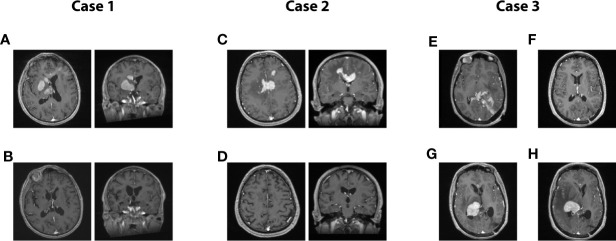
Representative cases from the series. (A, B): Case 1. A 71-year-old man presented with rapidly progressing left-sided hemiparesis combined with a sudden decline in cognitive functioning. On T1-gadolinium enhanced MRI, multiple nodular enhancements are obvious, devoid of significant oedema. Local mass effect can be observed on the ventricular system in axial and coronal acquisitions (A). Same MRI acquisition in axial and coronal after eight cycles of treatment (B). This patient remains disease-free to this date, 18 months after the conclusion of his last cycle. Clinically, he presented a full recovery. (C, D): Case 2. A 62-year-old man presented a gradual deterioration in cognitive functioning over a 2 months interval. Significant slowness in motor initiation and working memory were prominent features on presentation. A T1-enhanced MRI scan revealed multiple nodular enhancing lesions, mostly centered on the corpus callosum, and accompanied by oedema, in axial and coronal (C). T1-enhanced MRI scan in axial and coronal acquired at the conclusion of the treatment plan of 12 cycles, displaying a complete radiological response which was paralleled by a full clinical recovery (D). The patient remains disease-free 7 years after the conclusion of his treatment plan. (E–H): Case 3. A 42-year-old woman presented with mixed aphasia, confusion and severe headaches. What was considered confusion in retrospect was entirely attributable to a receptive speech deficit. She underwent an MRI scan which revealed a diffuse T1-enhancing mass localized in the left parieto-occipital area and diffusely infiltrating the splenium. The mass was partially resected in another center, before she started treatment with our team. The pre-treatment axial T1 MRI is shown in Panel (E), while the post-treatment MRI at the conclusion of eight cycles is shown in Panel (F). The patient was in complete response and had presented a full clinical recovery. She hence decided to stop treatment against our advice. Five months later, she presented with a left-sided deficit prompting an investigation. The T1-enhanced MRI revealed a relapse in disease in the contralateral hemisphere, as displayed on the axial T1-enhanced contrast MRI (G). She resumed treatment and responded for three cycles, only to present a massive progression before the initiation of the 4^th^ cycle (H). The patient then elected to discontinue all treatment, and she passed away 1 month after the relapse.
